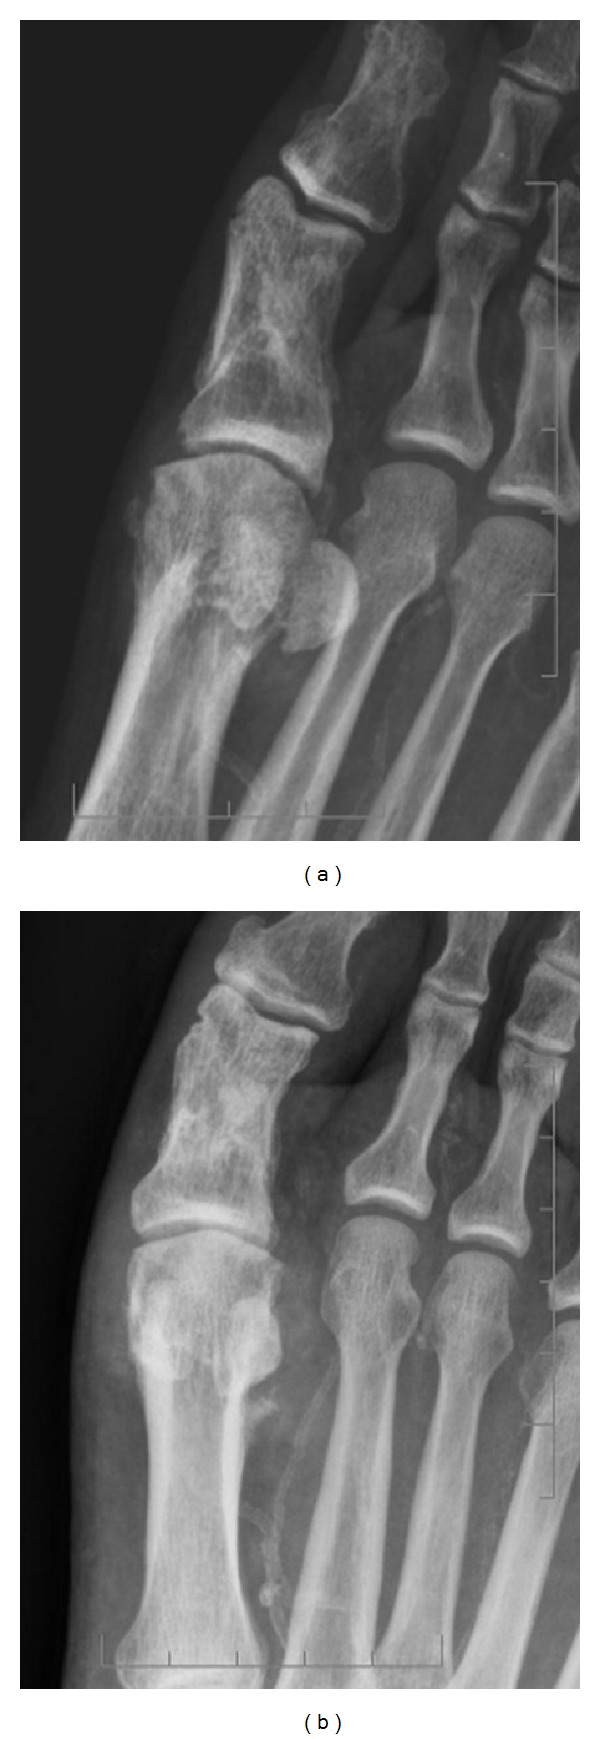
Initial radiographs show focal soft tissue reaction around the first MTP joint (emphasized by softer image windowing on (b)) without signs of bone affection. Healed fracture of the proximal first phalanx.
